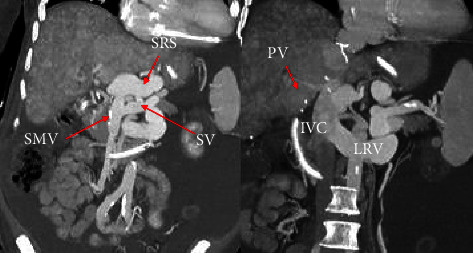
Contrast-enhanced CT showing both the large splenorenal shunt (SRS) draining the superior mesenteric vein (SMV) and splenic vein (SV) into the left renal vein (LRV) and the small transplant portal vein (PV) anastomosed to the inferior vena cava (IVC).
